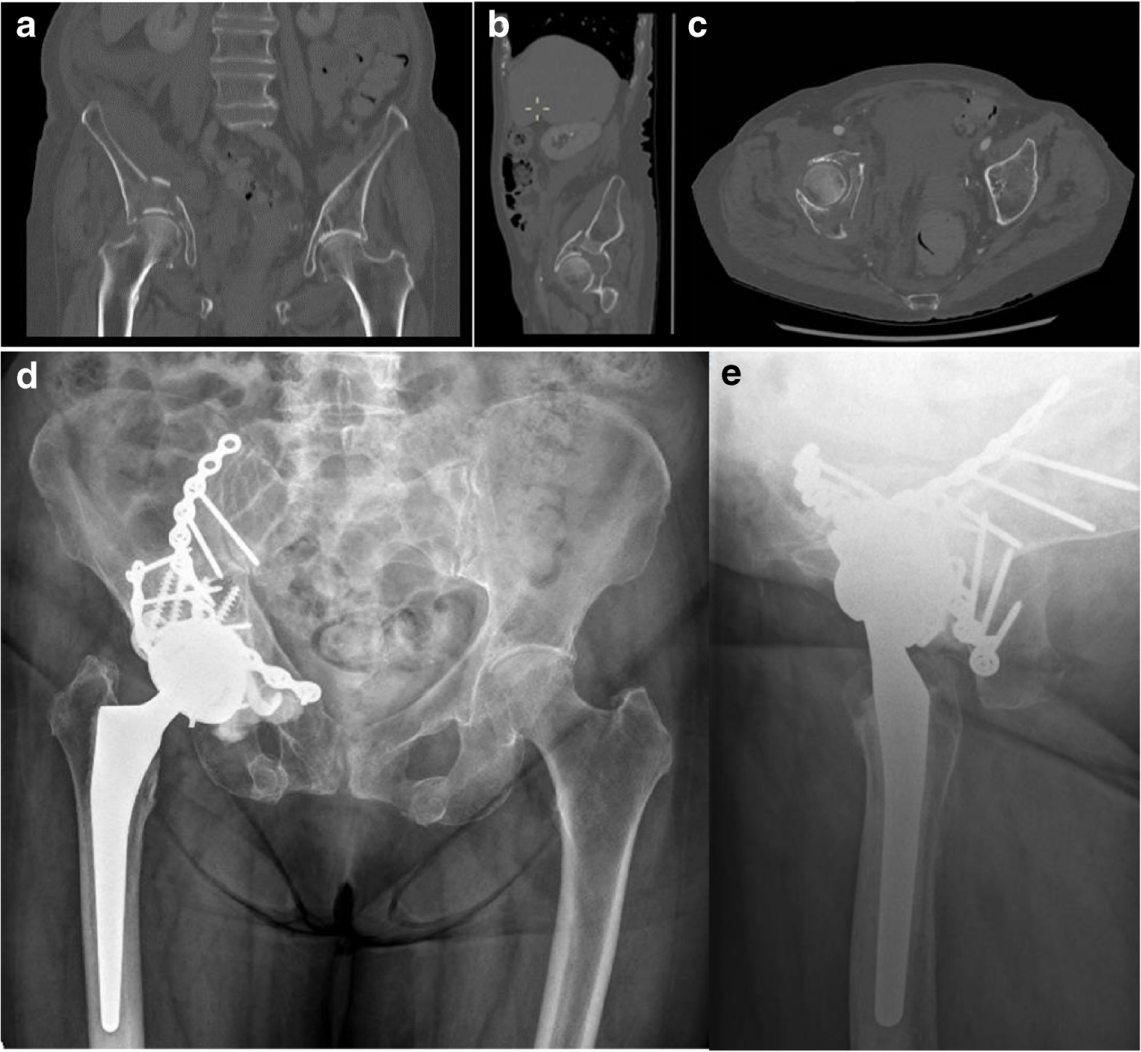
Pre-operative coronal, sagittal and axial views (a, b, and c) on CT scan of an 87-year-old female patient who sustained a both column fracture treated by DM-CHP with combined approach (CA-CHP). Anteroposterior pelvic (d) and axial hip (e) X-rays at 24 months of follow-up after CA-CHP showing implants in good position.
